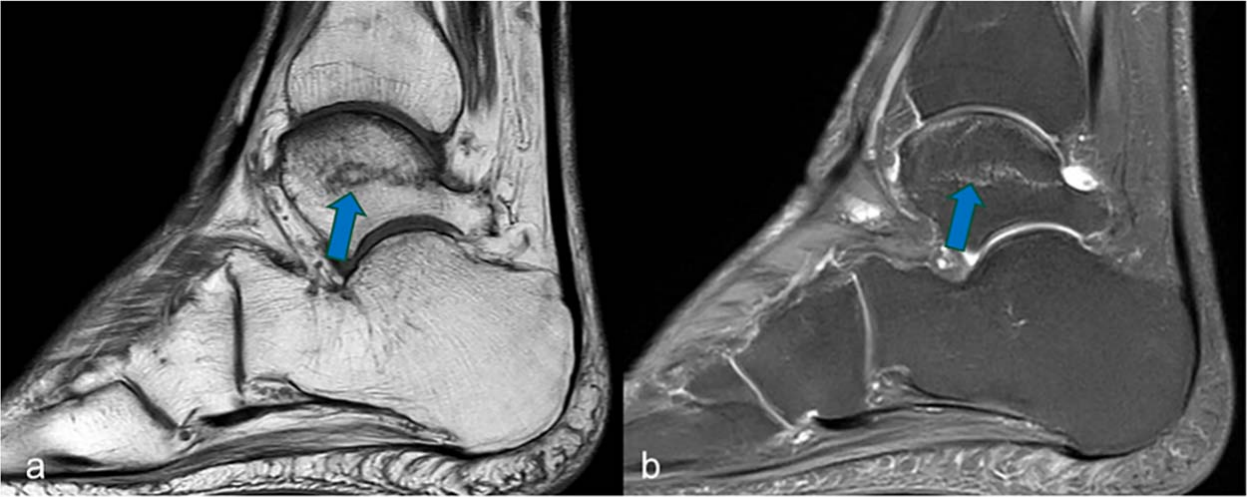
Sagittal MRI cross-sectional imaging showing (a) a T1-weighted and (b) a proton-weighted fat-suppressed image of the right tarsal bones. The ligamentous fracture in the trochlea tali was marked with arrows.
